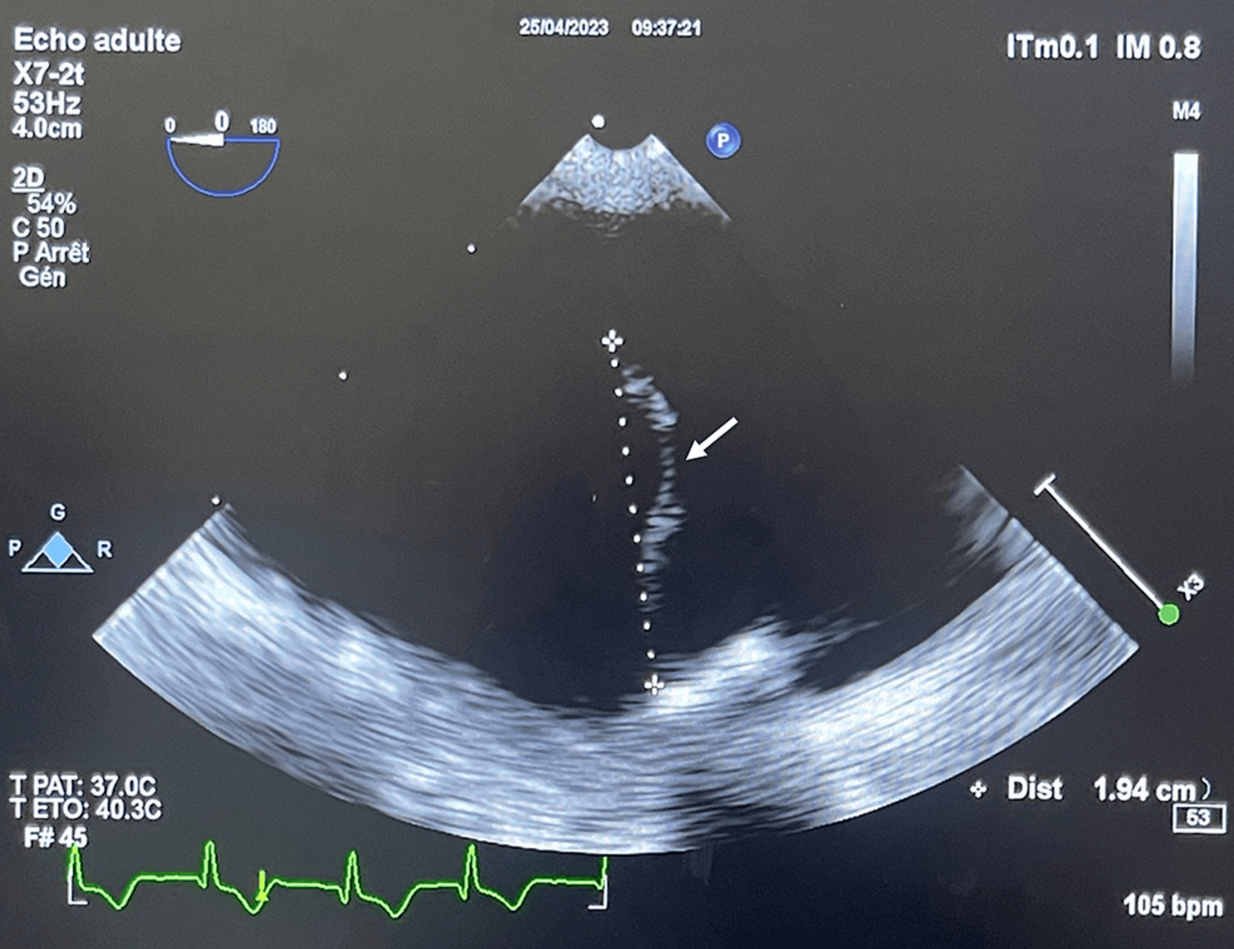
Mid-esophageal view on transesophageal echocardiogram showing serpentine vegetation affixed to the prosthetic valve
